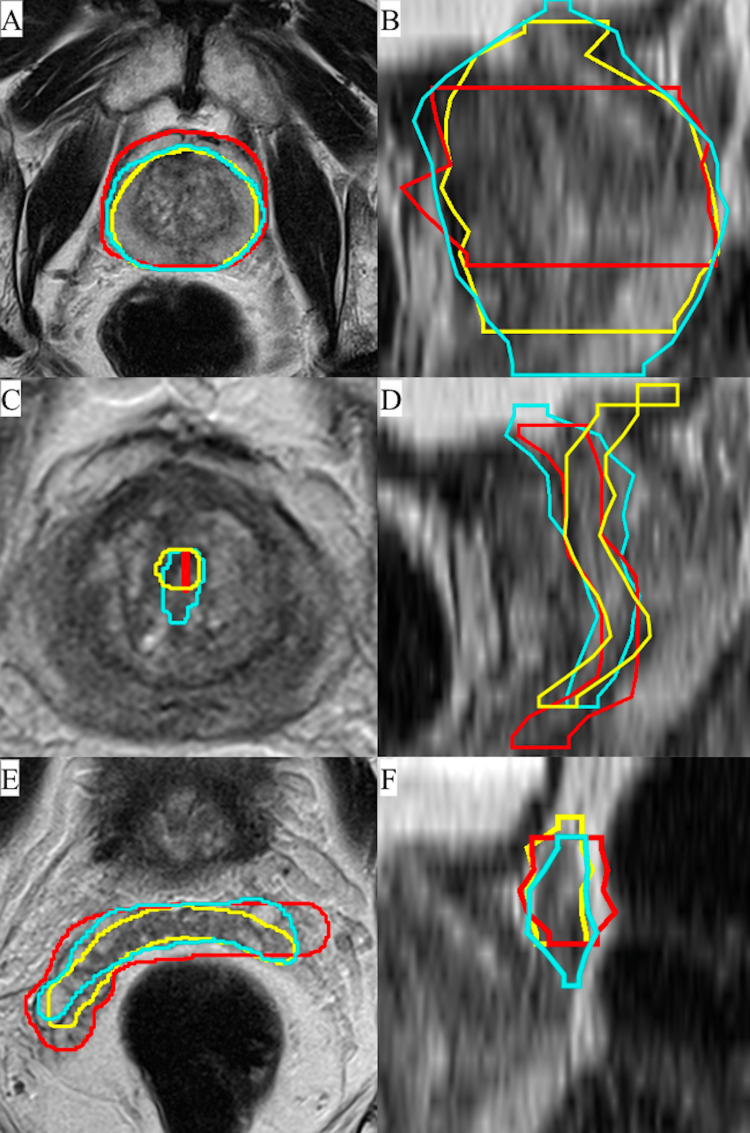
Representative T2-weighted prostate MRIs demonstrating AI-, urologist-, and radiologist-generated contours: (A) axial view of the prostate, (B) sagittal view of the prostate, (C) axial view of the urethra, (D) sagittal view of the urethra, (E) axial view of the seminal vesicles, and (F) sagittal view of the seminal vesicles AI-generated contours are shown in cyan, urologist contours in yellow, and radiologist contours in red. AI: artificial intelligence; MRI: magnetic resonance imaging
